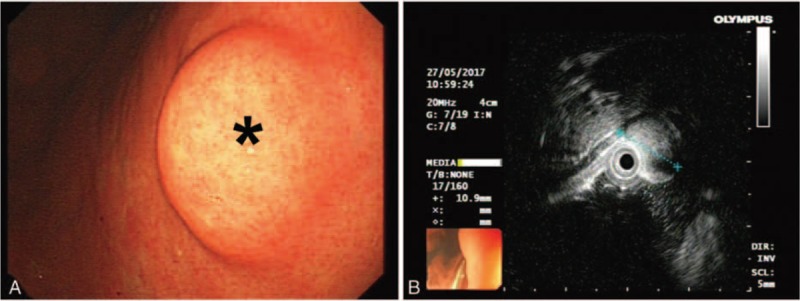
Gastroscopy indicated a semi-circular submucosal neoplasm of 2 cm on the posterior wall (as shown by the asterisk) of the stomach (A). Endoscopic ultrasonography showed a low-density lesion with distinct boundary, which was derived from the muscularis propria of the gastric wall (B).
